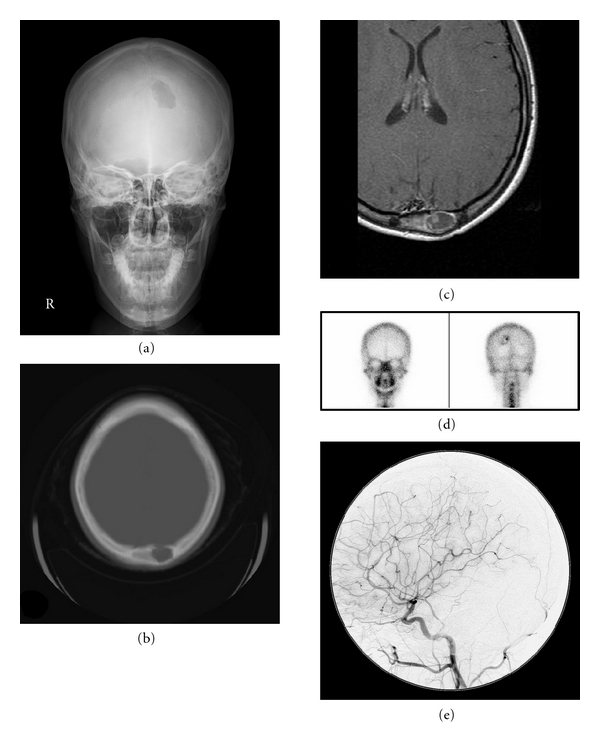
AP view of skull xp (a) showing the bony defect of the left occipital lesion. Bone image CT at the level of the subcutaneous mass (b) demonstrating the bony defect of the left occipital bone without a change in the intraaxial lesion. The skull surface of the defective part was swollen. Axial T1-weighted MRI with contrast medium (c) showing a cystic change corresponding to the bony defect and partial enhancement around the cystic change. ^99m^Tc-HMDP bone scintigraphy (d) showing accumulation of tracer in the part of the bone cyst corresponding to the strongly enhanced lesion found on MRI. Head DSA study (e, lateral view) demonstrating no abnormal vessels, including tumor feeder or aneurysmal changes.
